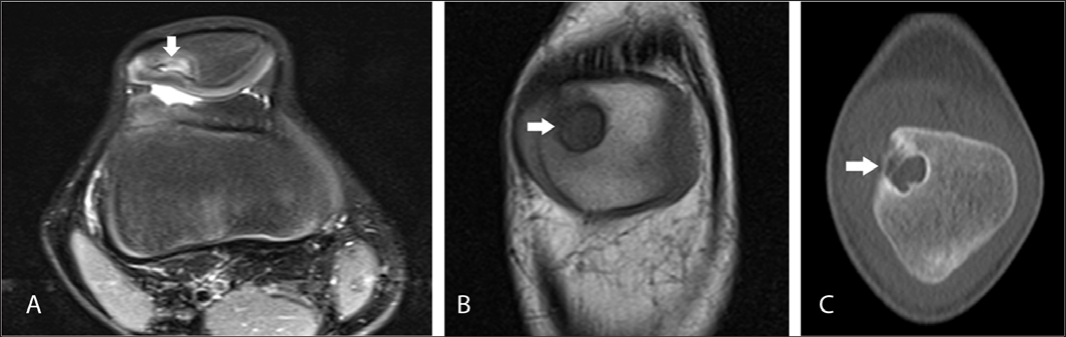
Dorsal defect of the patella. Axial FS T2-WI (A) and coronal T1-WI (B) show a well-delineated defect at the superolateral aspect of the right knee (arrow). The overlying articular cartilage is intact. On cone-beam CT (C), the defect is sharply delineated and has a sclerotic border (arrow).
